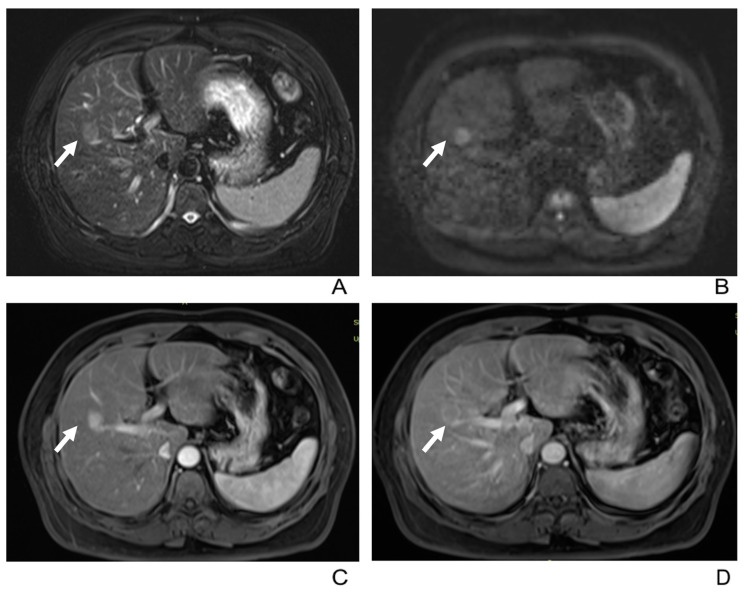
52-year-old male with intrahepatic lymphoepithelioma-like cholangiocarcinoma. (A) T2-weighted MR image displays a well-defined hyperintense tumor (arrow) in the right lobe of the liver. (B) Tumor (arrow) shows hyperintensity on diffusion-weighted imaging (b = 500 m^2^/s). (C,D) Tumor (arrow) shows “rapid wash-in and rapid wash-out (Type B enhancement pattern) in the arterial and portal venous phase and delayed capsular enhancement in the portal venous phase.
